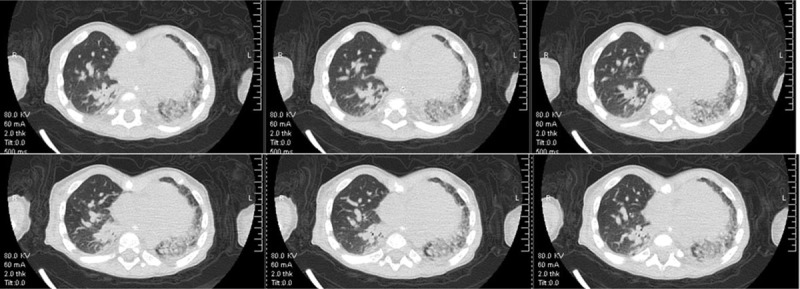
The computed tomographic (CT) scan on the 17th hospitalization day (illness day 23). Multiple ground-glass opacity and patchy and flaky high-density shadows in both lungs, enlargement of hilar shadows and thickened pulmonary vessels.
